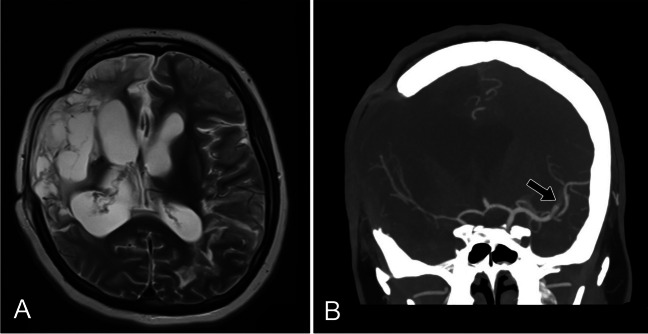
A MRI T2 showing encephalomalacia, B CTA MIP showing thromboembolic occlusion (black arrow) of the M2 branch of the left MCA
